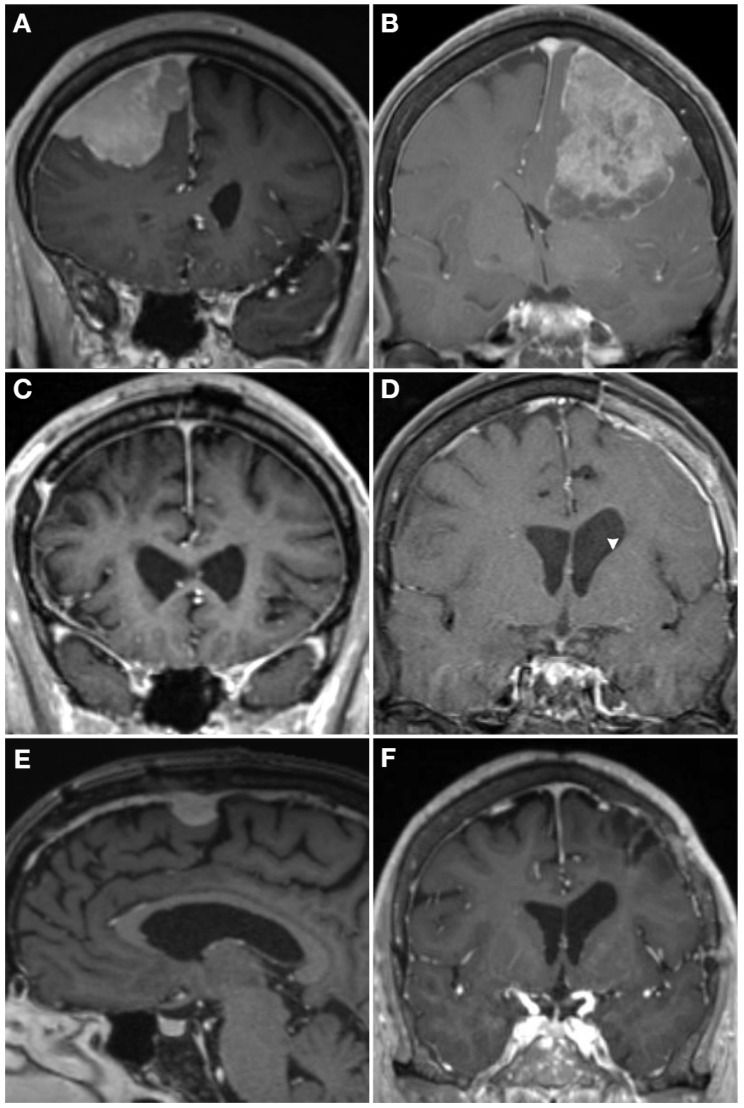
Case examples demonstrating how molecular profiling can inform care. T1 post-contrast MRI from two WHO grade 2 meningiomas (A,B) who both presented with a seizure and underwent gross total resection (C,D). The patient on the left had a hypermitotic methylation profile and chromosome losses at 1p, 6, 14, haplo-insufficiency of CDKN2A, and 22q, making it a Driver et al. integrated grade 3 tumor, with a high Chen et al. gene expression risk score. He underwent 59.4 Gy adjuvant radiotherapy and had an in-field recurrence (E) at 15 months post-operative. The patient on the right also had a WHO grade 2 meningioma that had an immune-enriched methylation profile and chromosome 8 loss with haplo-insufficiency of chromosome 22, making it a Driver et al. integrated grade 1 tumor [86], and had a low Chen et al. gene expression risk score [126]. She was observed given the favorable molecular profile without adjuvant radiotherapy and had no recurrence at 2 years post-operative (F).
